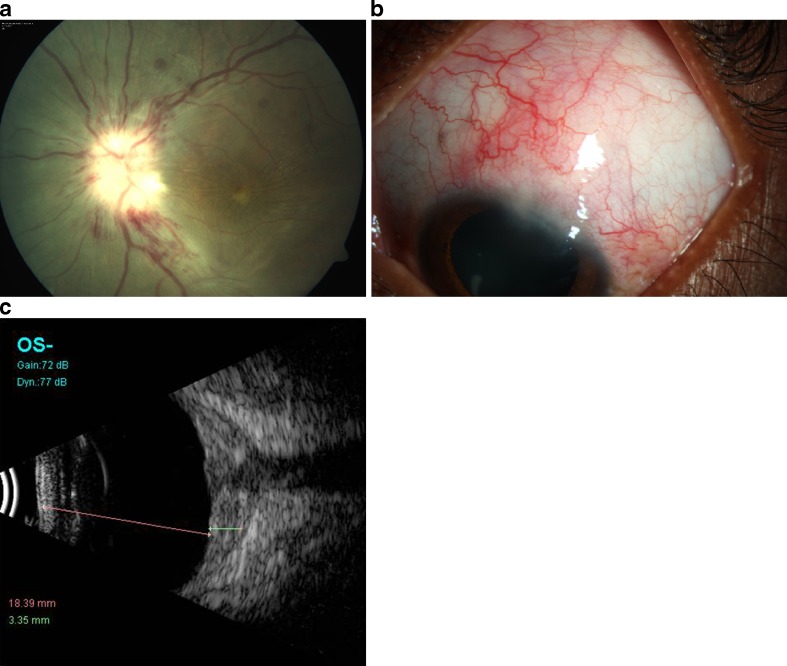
a Fundus photo at 1 week posttreatment showing resolving exudative detachment and resolving hemorrhages. b Anterior scleritis with mild resolution at 1 week posttreatment. c Ultrasound B scan showing decreased thickness of the sclera and choroid and reduction in the “T” sign
